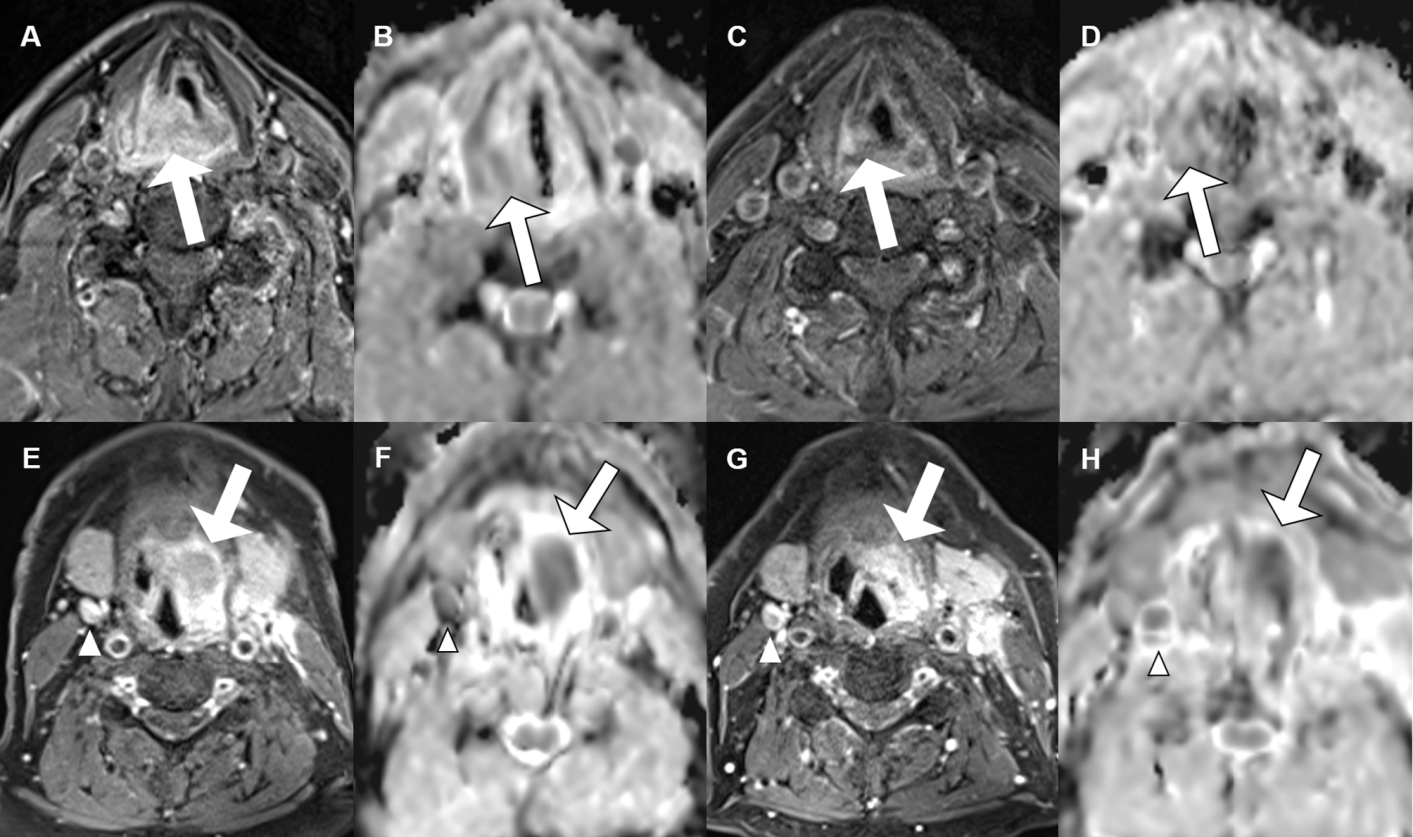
Morphological and functional MRI images for two patients with pharyngeal carcinoma (primary tumor is marked with an arrow, lymph node metastasis with an arrow head), contrast enhanced T1 weighted GRASP-VIBE sequence (A, C, E, G) and ADC map (B, D, F, H) for both a patient with pathologic complete response (upper row) and a patient with residual tumor (lower row) at baseline [(A, B) and respectively (E, F)] and at follow-up [(C, D) and respectively (G, H)].
